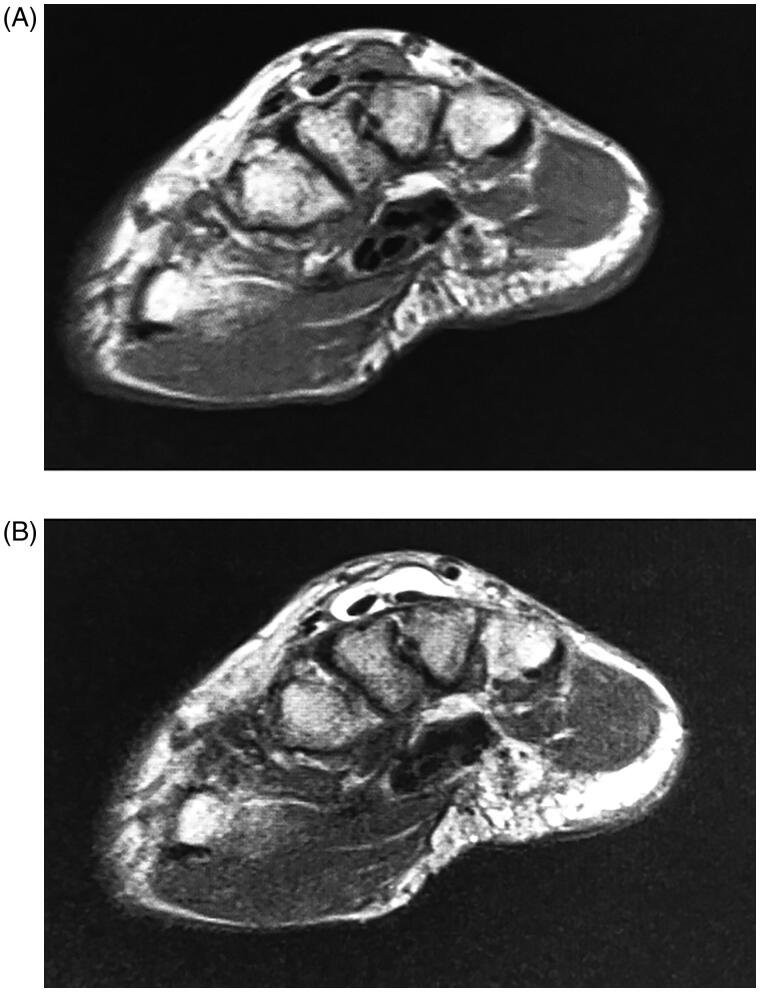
Preoperative axial MRI. At the metacarpal bone base level, lesions of approximately 8.3mm around the 3rd extensor digitorum communis (EDC) tendon and 11.3 mm around 4th EDC tendon were noted. Each showed a low-intensity lesion on T1-weighted imaging (A) and a high intensity on T2-weighted imaging (B).
